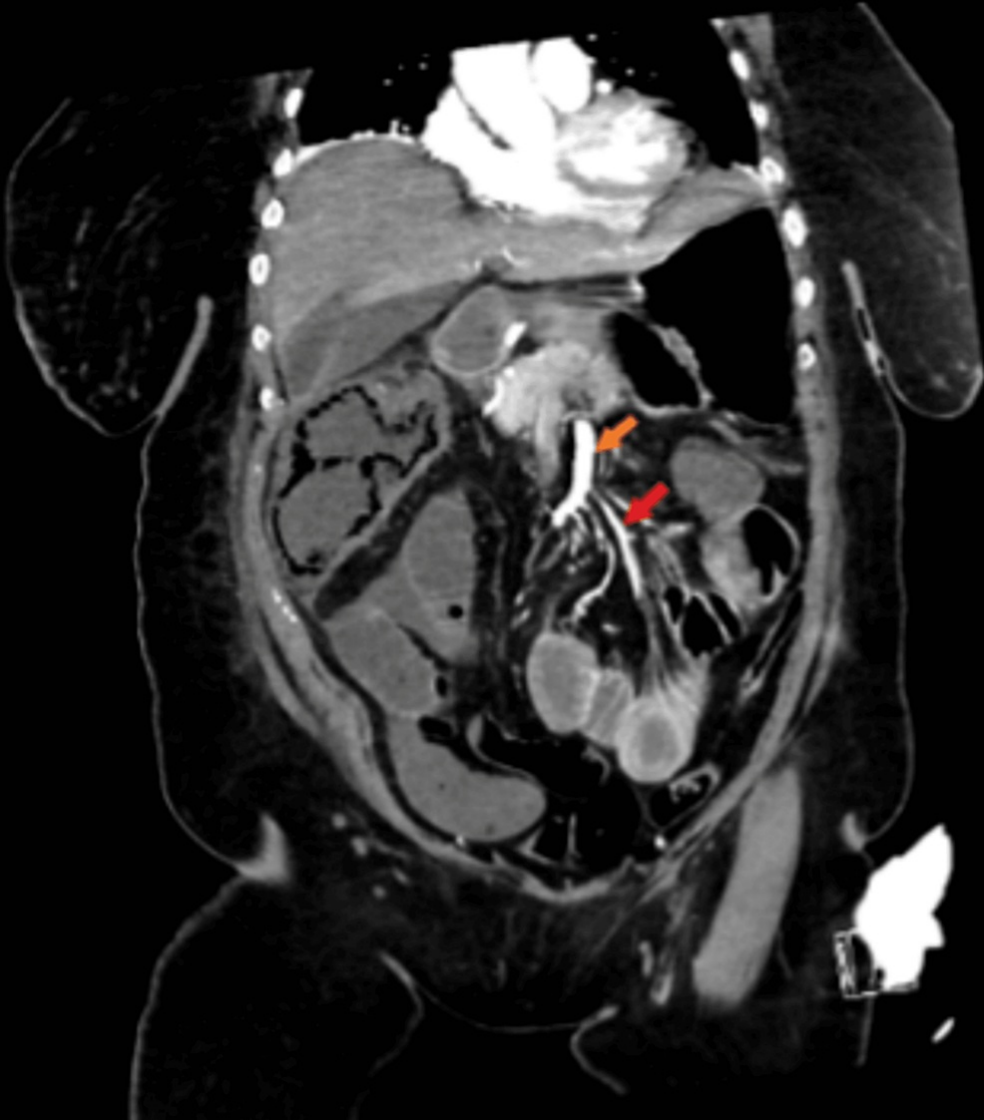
Coronal section of CT angiogram abdomen and pelvis showing descending aorta with occlusion (orange arrow), and inferior mesenteric artery (IMA) with occlusion (red arrow).
